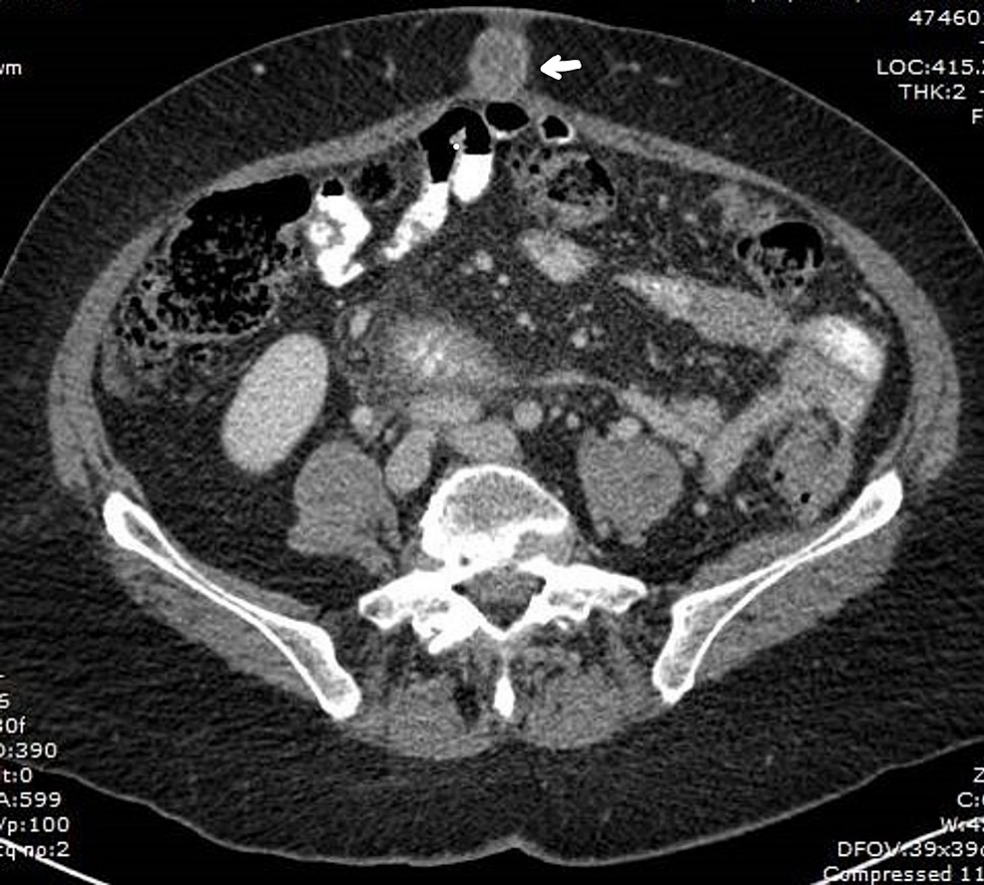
CT scan showing umbilical lesion
